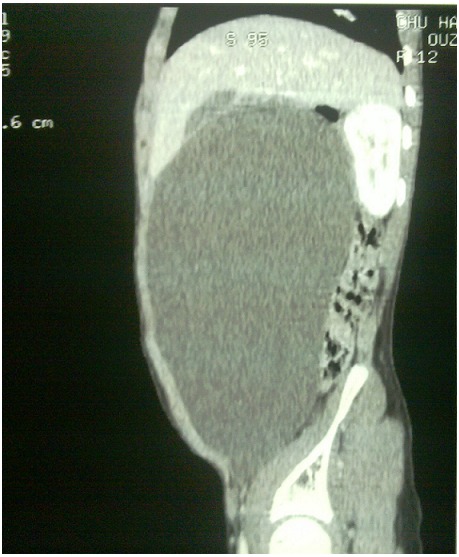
Sagittal view of the abdominal CT scan of the third patient, demonstrating a huge cystic mass
